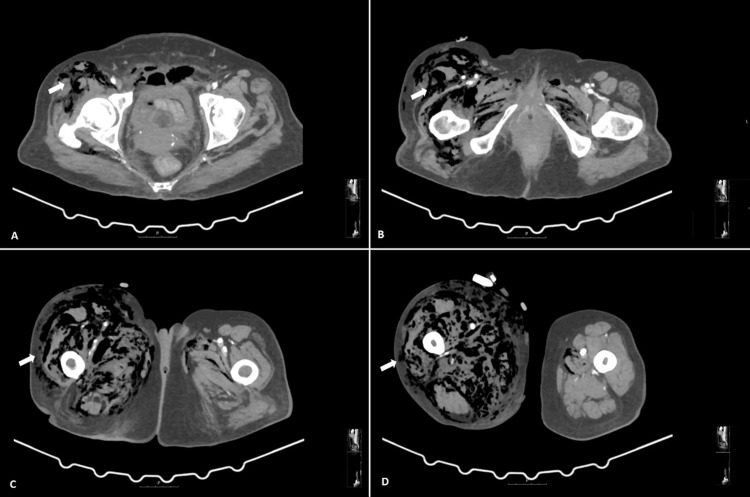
Axial CT images of the pelvis and thighs (A–D) Axial CT slices demonstrating extensive subcutaneous and intermuscular emphysema of the bilateral anterior abdominopelvic wall, with gaseous foci extending to the right iliopsoas muscle and both thighs (white arrows), consistent with NF.
